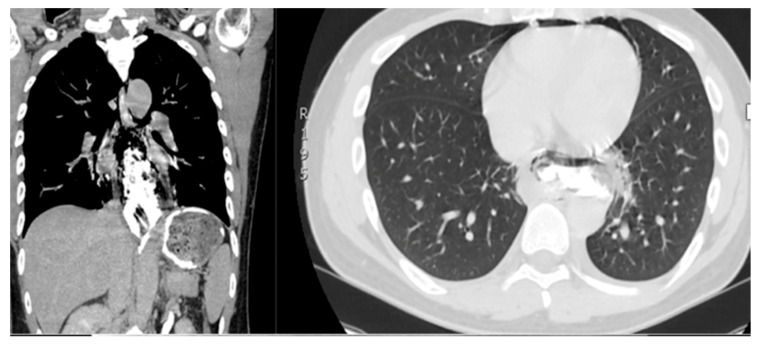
CT scan showing oesophageal perforation after ingestion of FB.
